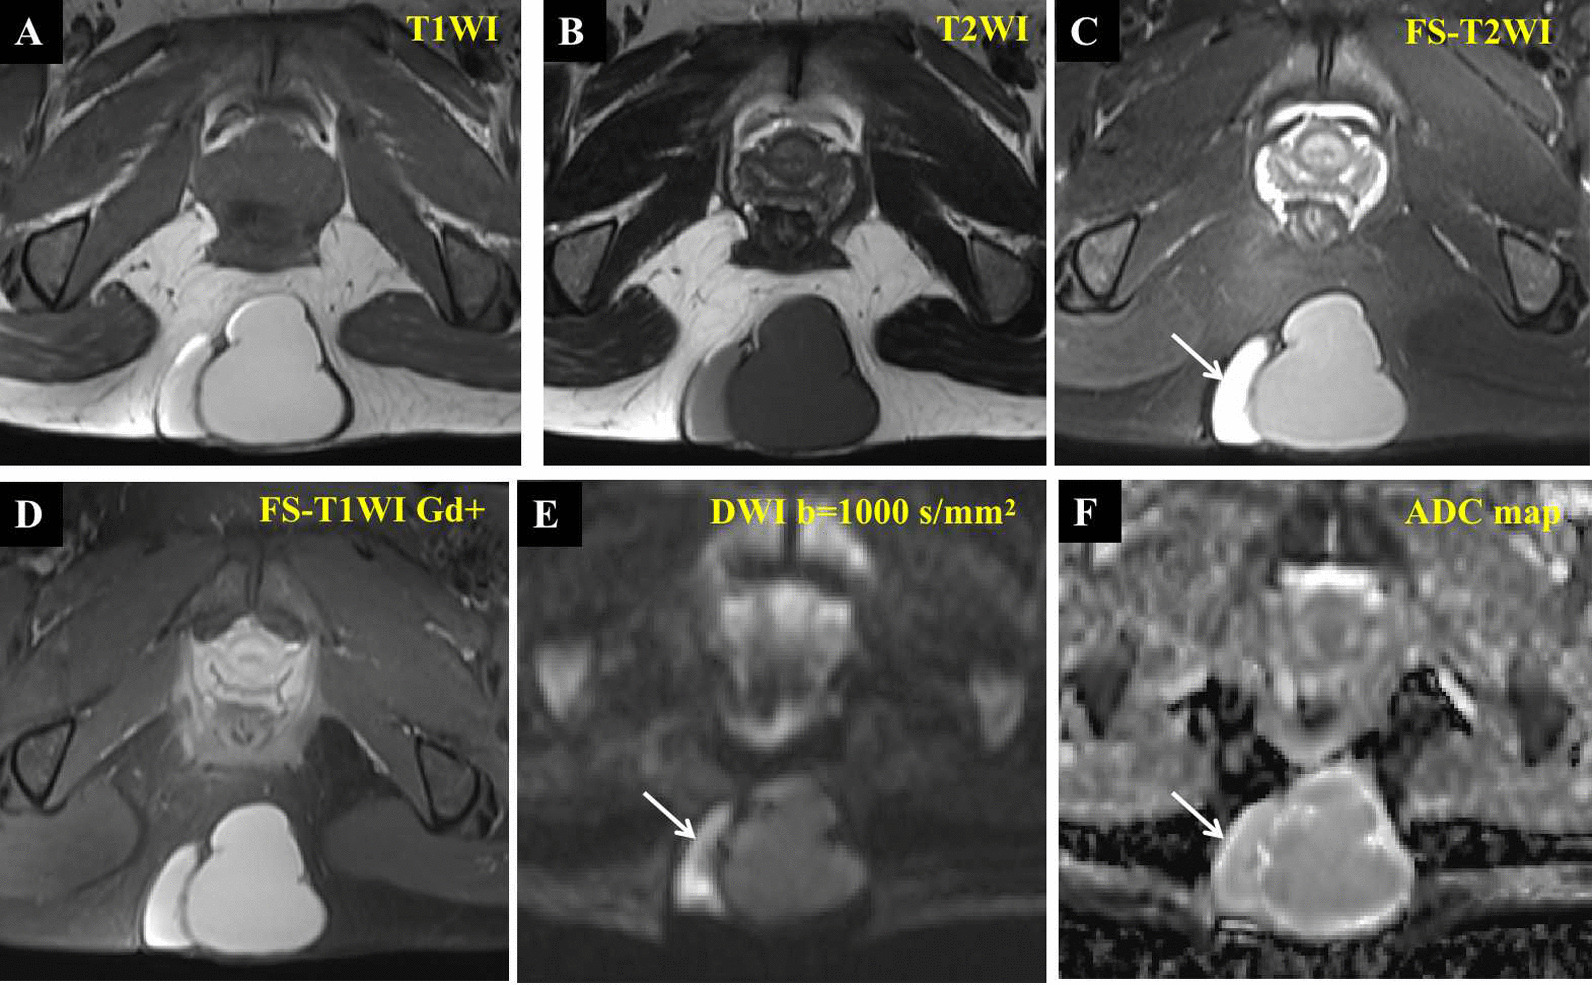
Preoperative magnetic resonance imaging. Axial T1 weighted image (A), T2 weighted image (B) and fat-sat T2 weighted image (C) show a cyst of similar dimensions with heterogeneous hyperintensity. Fat-sat post contrast T1 weighted image (D) reveals no contrast enhancement or evidence of solid components. (E, F) Axial diffusion-weighted images show signals of heterogeneous isointensity and hyperintensity (E, arrow) due to a T2-shine-through phenomenon (C, arrow) without restricted diffusion foci (F, arrow)
